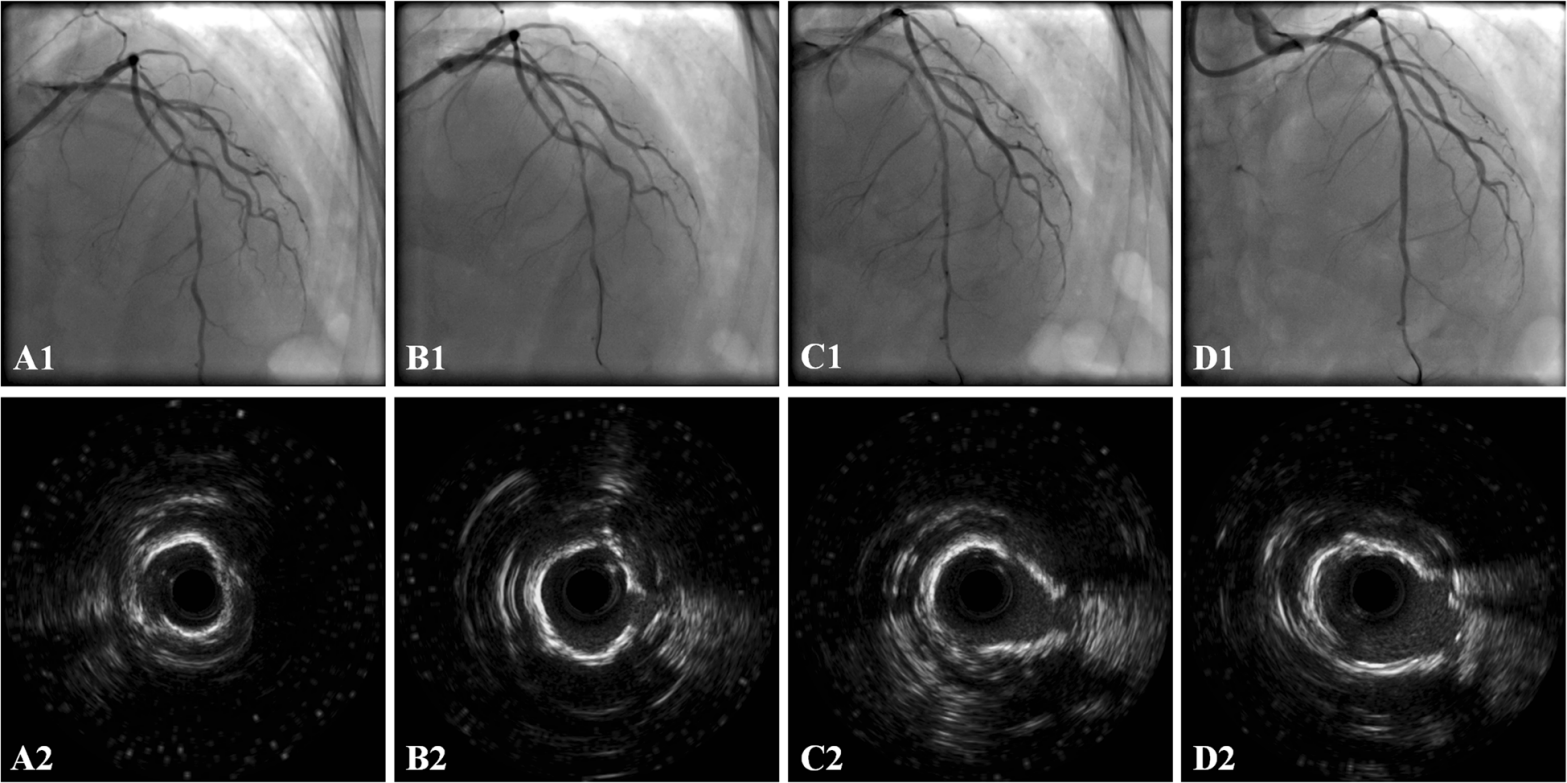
The angiography and intravascular ultrasound (IVUS) images of severe coronary calcification lesion before and after rotational atherectomy, cutting balloon, and stent deployment. A1 and A2, Severe calcific stenosis of the *left* anterior descending artery; B1 and B2, post-rotablator; C1 and C2, post-cutting balloon; D1 and D2, post-stent
